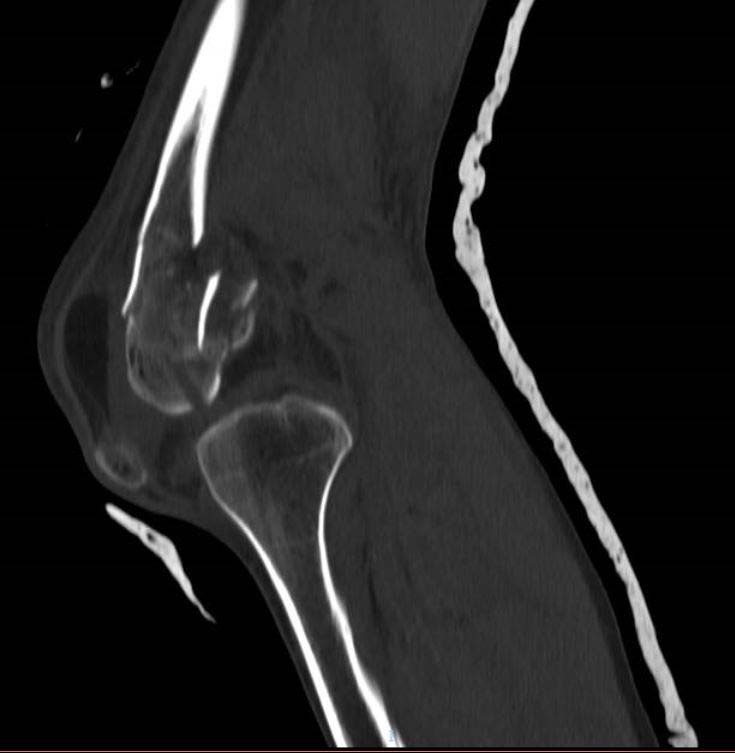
Use of CT Scan to determine fracture configuration, size of bony comminution and bone stock; Sagittal Section.
